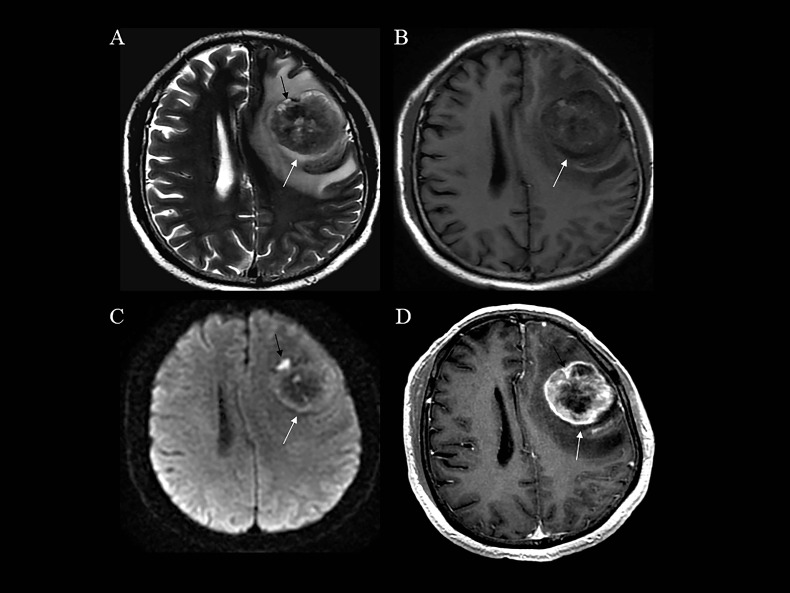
Role of DWI and contrast-enhanced T1WI in the identification of the pool sign. A perilesional T2-Weighted Imaging (T2WI) hyperintense rim (A, B white arrow) was observed between the lesion and the surrounding edema, without diffusion restriction (C, white arrow) and without enhancement (D, white arrow). This region demonstrated a true pool sign. Conversely, a similar T2WI hyperintense region was observed (A, black arrow), but with diffusion restriction (C, black arrow) and enhancement (D, black arrow). This corresponding area exhibited a false pool sign.
